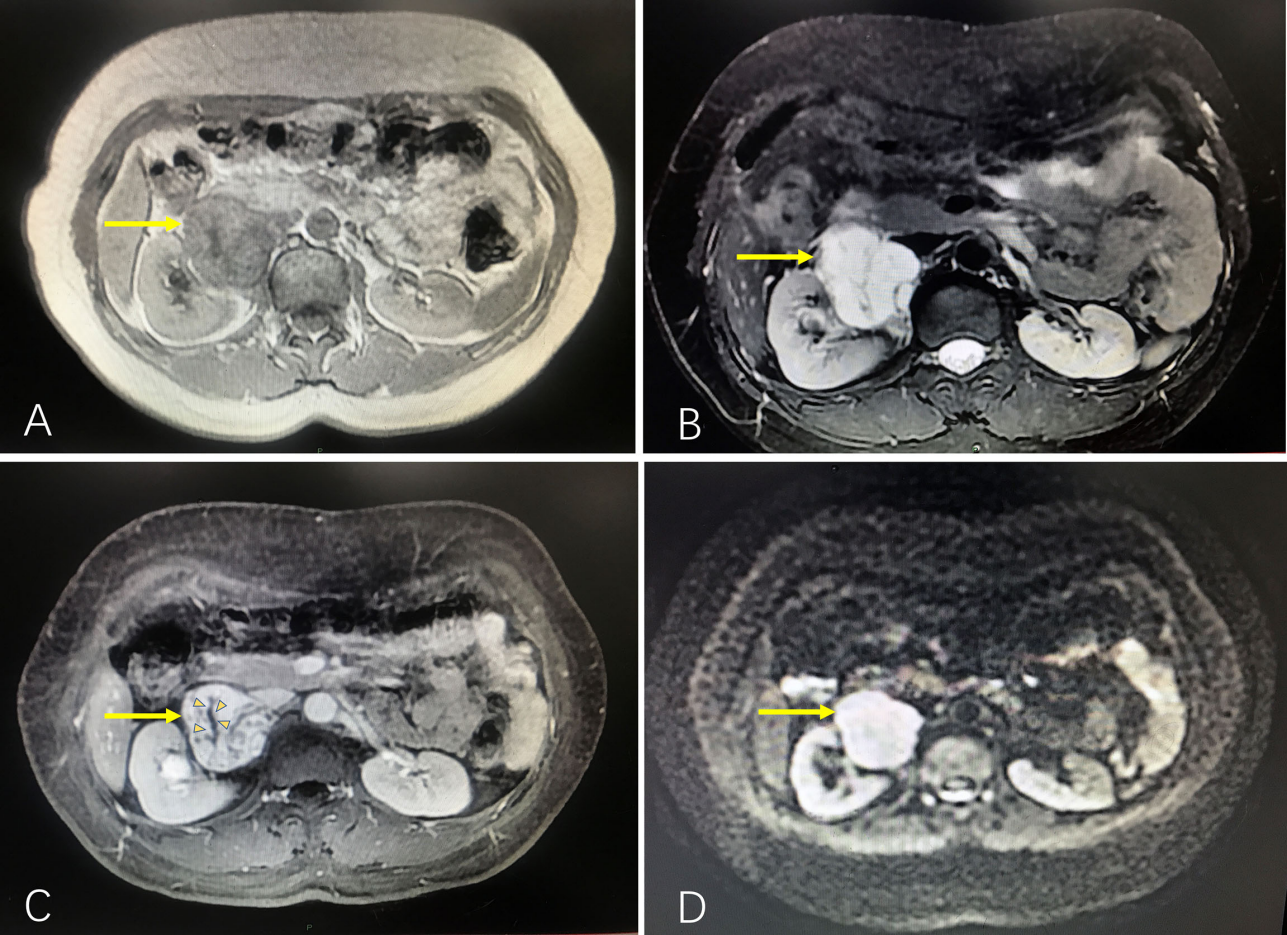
Abdominal MRI showing the mass compressing the right renal pelvis and ureter, and the right renal arteriovenous and inferior vena cava were also slightly compressed. (A) T1WI, low signal similar to the abdominal aorta (arrow). (B) T2WI, high signal for tumor (arrow). (C) Contrast-enhanced scan revealing that the tumor had an obvious enhancement with a central hypointense area (small arrow heads), and the filling of the contrast agent from the edge to the center was delayed. (D) DWI, high signal for the tumor (arrow).
